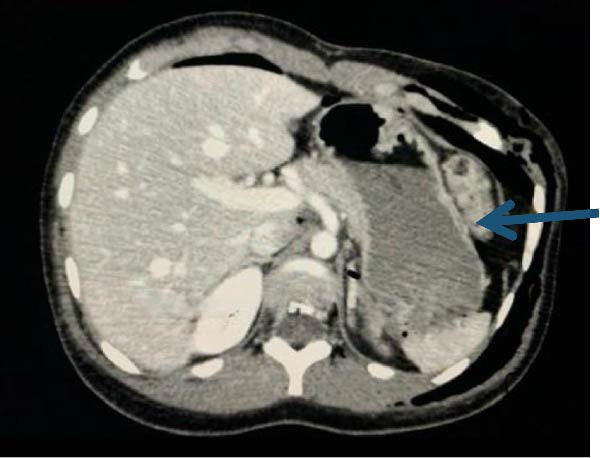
Axial computed tomography of the abdomen. The blue arrow shows the upward displacement of intestinal loops, suggesting hemidiaphragmatic rupture. *Source:* Image obtained during the study.
